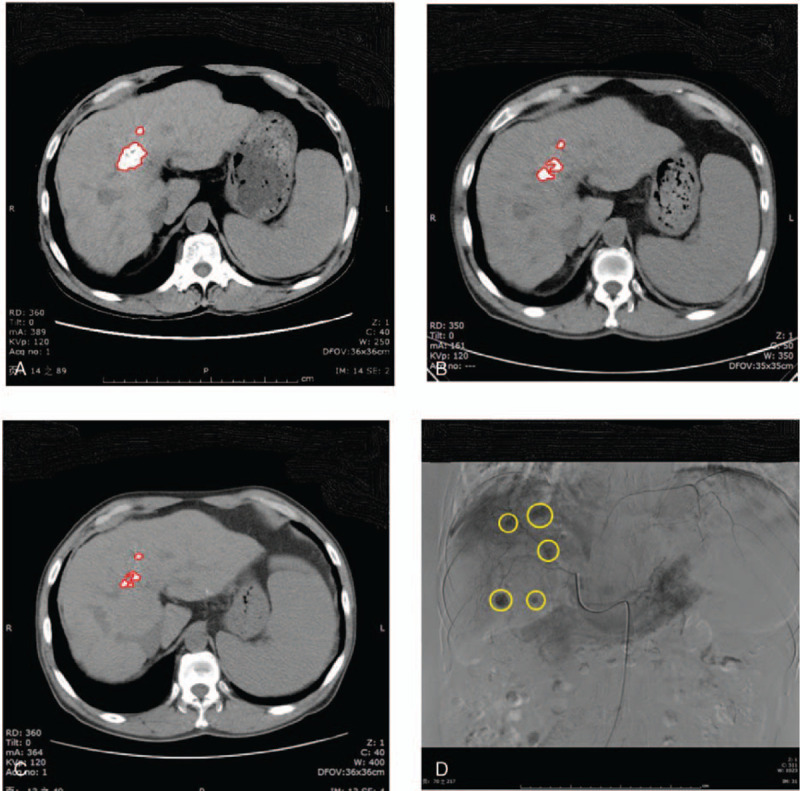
Liver images from case 1 A. After the first TACE (December 18, 2018), the liver plain CT scan showed that the liver had multiple high-density shadows. B. After the second TACE (March 19, 2019), the liver plain CT scan showed that some of the high-density shadows in the liver were smaller than before (December 18, 2018). C. After the third TACE (November 27, 2019), the liver plain CT scan showed that some of the high-density shadows in the liver were smaller than before (March 19, 2019); D. The fourth TACE (November 29, 2019) angiography showed the superior deposition of lipiodols. The circles indicate the tumors.
